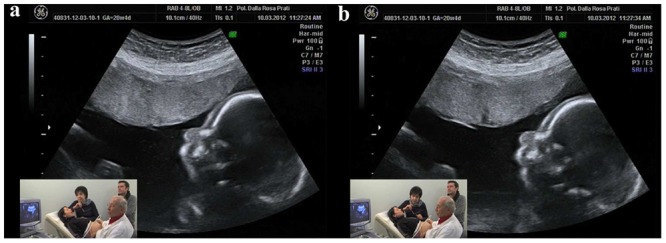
An ultrasonographic image and the experimental setting. The small frame shows the mother and ecographer, together with the operator (AFG) and the father. (a) Shows the mother while opening the mouth and (b) shows the fetus’ congruent response of mother’s mouth opening.
